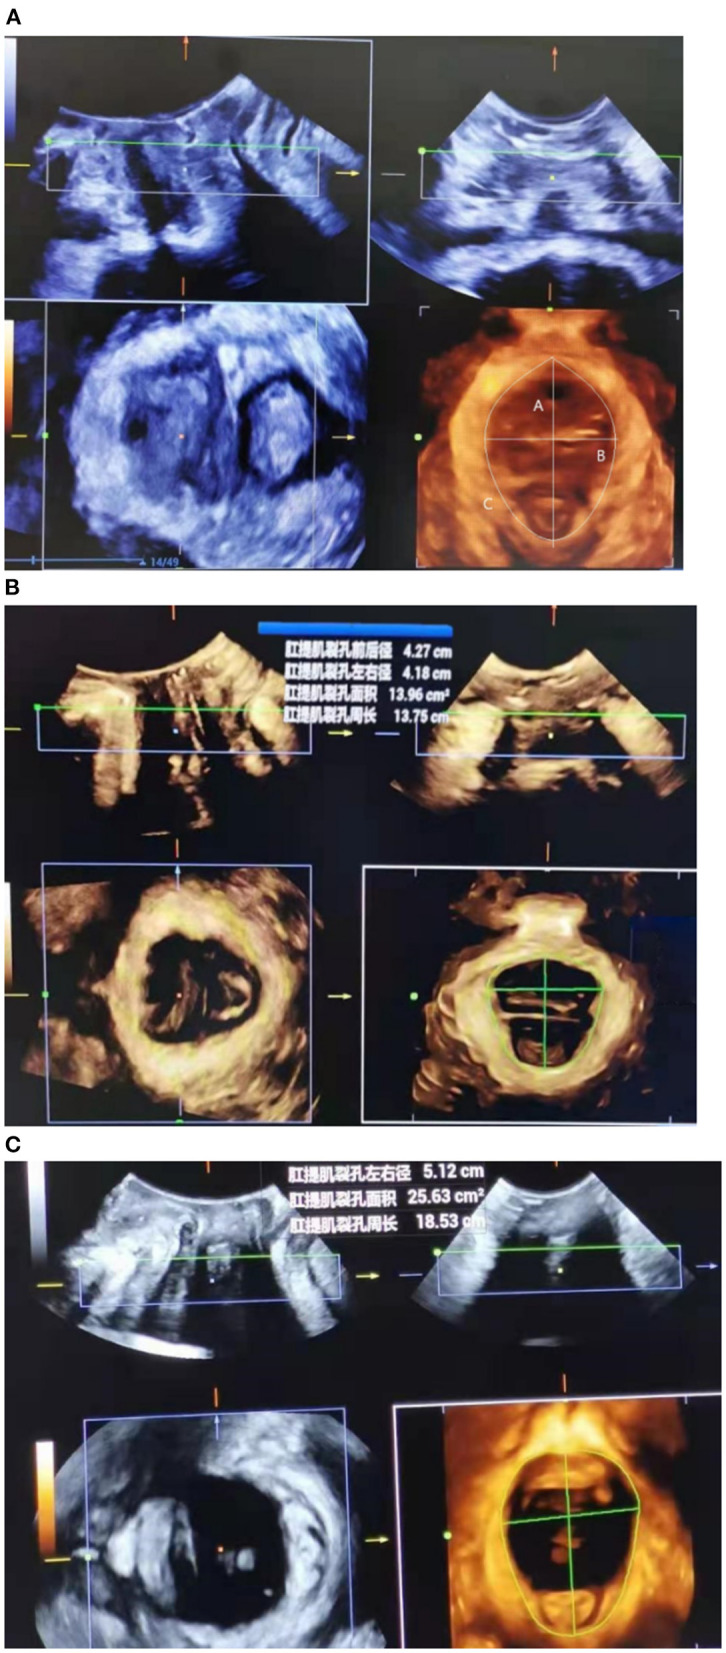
Transperineal ultrasound image demonstrating the measurement of levator hiatal anteroposterior diameter (upper left), left-right diameter (upper right), and area (lower left) at rest (A), at maximal contraction (B), or under Valsalva maneuver (C).
